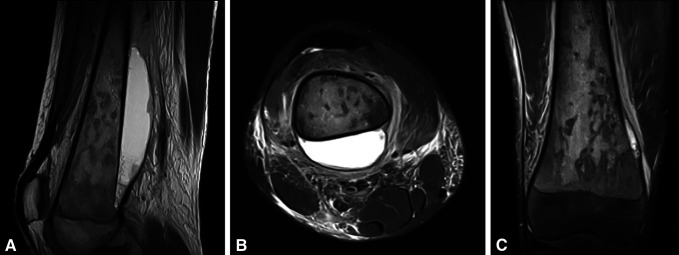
MRI of the right knee. Distal femur sagittal (A) and transverse (B) views, which demonstrate the large fluid collection on the posterior surface of the distal femur and diffuse soft-tissue edema. Distal femur coronal (C) view, which shows bone marrow heterogeneity and elevated metaphyseal periosteum.
